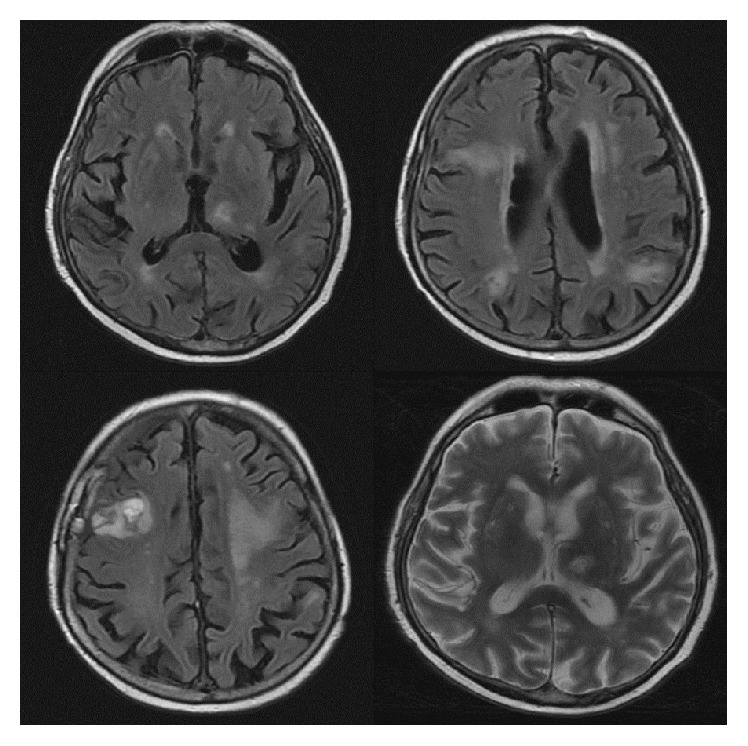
MRI after completion of rituximab and whole brain radiation (day 97). Rituximab started on day 63; cranial XRT started on day 70. Abnormal parenchymal enhancement in both cerebral hemispheres that have decreased in size. Increased hemorrhage in bilateral frontal and parietal lobe lesions. In the right frontal lobe there is a 15 mm rim-enhancing fluid collection. The edema surrounding most of the lesions has decreased in size. The left cerebellar lesion has decreased from 5 mm to 2 mm.
